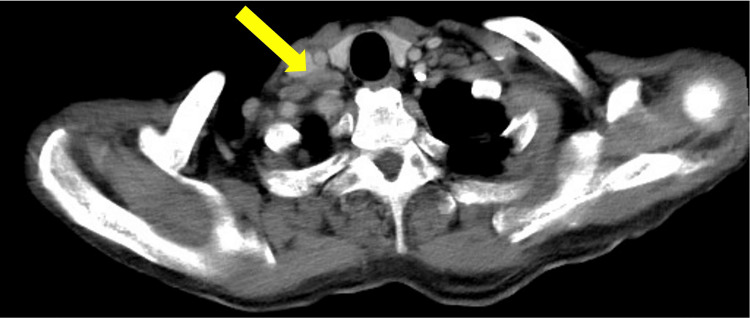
Contrast-enhanced CT of the chest and pelvis showing infraclavicular lymphadenopathy. CT revealed right supraclavicular lymphadenopathy (arrow) with peripheral heterogeneous enhancement and central low attenuation.
